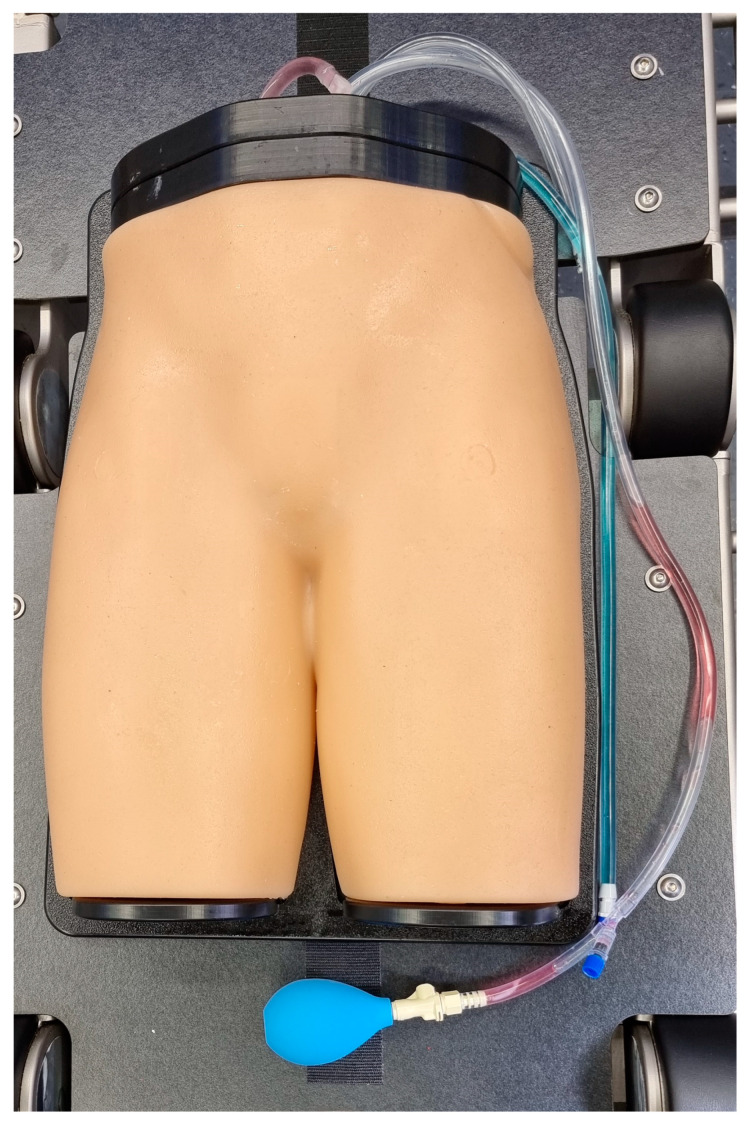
Gen II Femoral Vascular Access and Regional Anesthesia Ultrasound Training Model (CAE Healthcare, Mainz, Germany).
